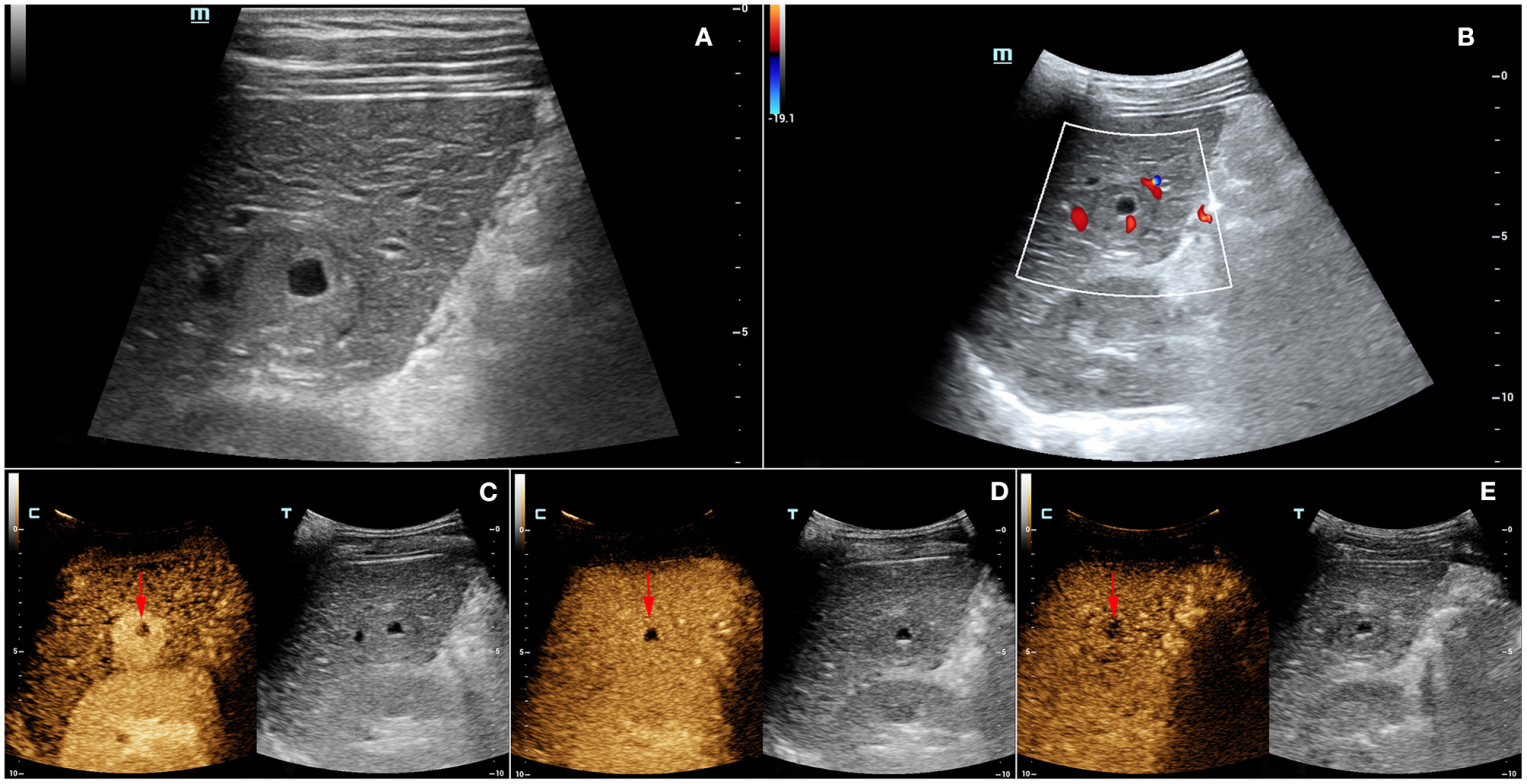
(A) Grayscale ultrasound showed a hypoechoic nodule with peripheral hypoechoic halo and centrally anechoic area in the hepatic segment VI. (B) Color Doppler flow imaging (CDFI) displayed dot-linear blood flow signals within the liver nodule. In the contrast-enhanced ultrasound (CEUS), (C) the solid component of the nodule presented rapidly homogeneous hyper-enhancement in the arterial phase. (D) The enhancement of the nodule was washed out slowly and gradually, with heterogenous mild enhancement in the portal phase. (E) In the late phase (4 min after contrast agent injection), the nodule showed slight hypo-enhancement, and the hypo-enhanced area was mainly located in the surrounding space. There was a small piece of non-enhanced area (arrows) within the lesion center in the whole three-phase imaging.
